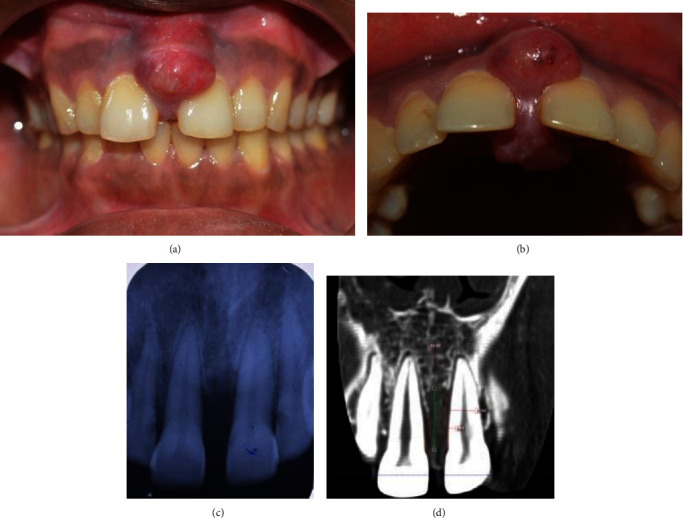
(a) and (b) Dumbbell-shaped swelling involving the buccal and palatal aspect. (c) Intra-oral peri-apical radiograph showing interdental bone loss between maxillary central incisors; (d) CBCT showing bone loss in the mid-alveolus region.
